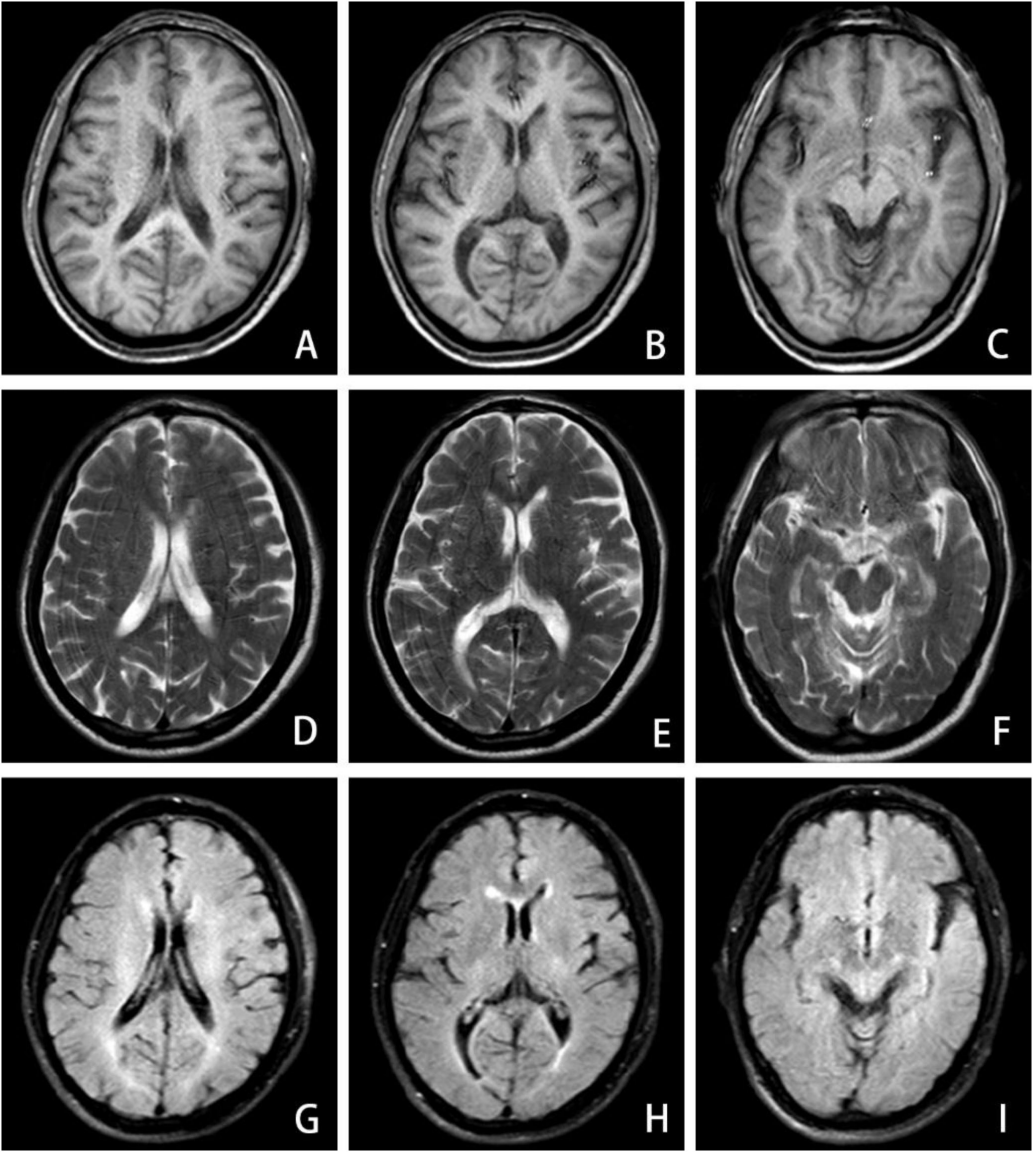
MRI brain imaging exhibits demyelinating-like alterations in the anterior and posterior horns of the lateral ventricles across both cerebral hemispheres, including T1-weighted (A–C), T2-weighted (D–F), and T2-FLAIR (G–I) sequences.
